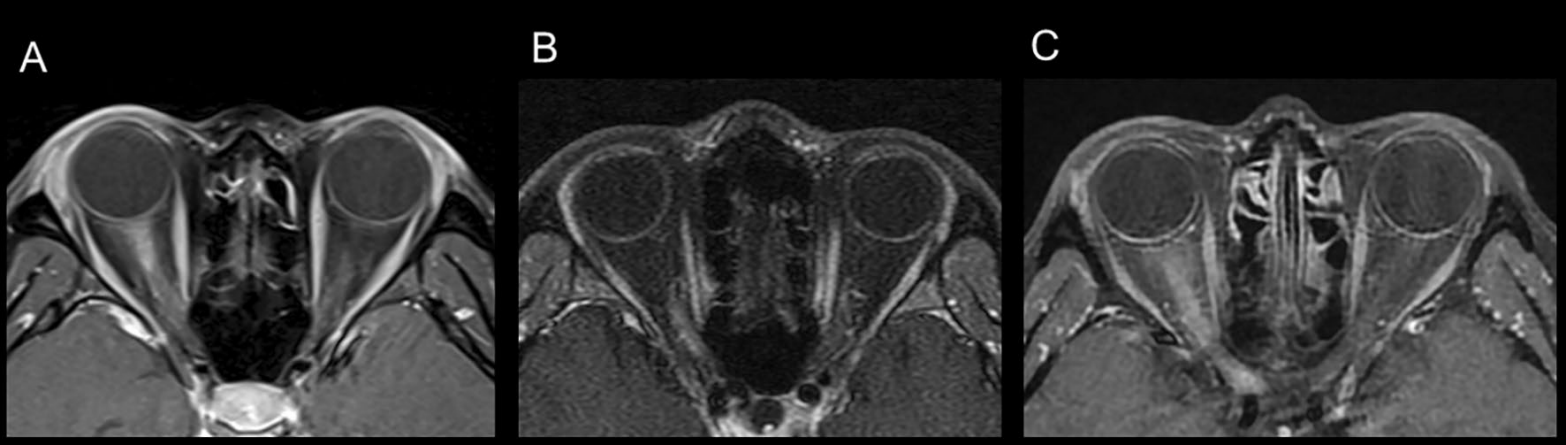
Location of abnormal findings on MRI. (A) intraorbital lesion, (B) intracanalicular area, (C) whole optic nerve involvement (optic nerve to canal).
